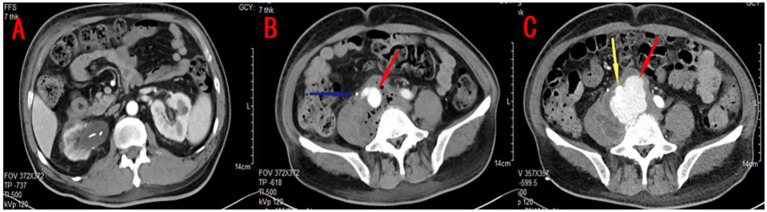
Contrast-enhanced CT findings: (A) The boundary between the lesion and the psoas major muscle, as well as the blood vessel, was unclear. A right common iliac aneurysm (red arrow), approximately 2.2 cm in diameter, and a right ureteral stent (blue arrow) were observed. (B) The right kidney appeared to be atrophied, irregularly shaped, and surrounded by mild inflammation. (C) Follow-up contrast-enhanced CT showed that the enhancement shadow around the iliac artery (yellow arrow) had significantly increased in size compared to the previous scan and exhibited an irregular shape. A mass-like shadow (red arrow) with a density lower than that of arterial blood vessels was observed, suggestive of rupture of the right common iliac aneurysm with localized encapsulation.
